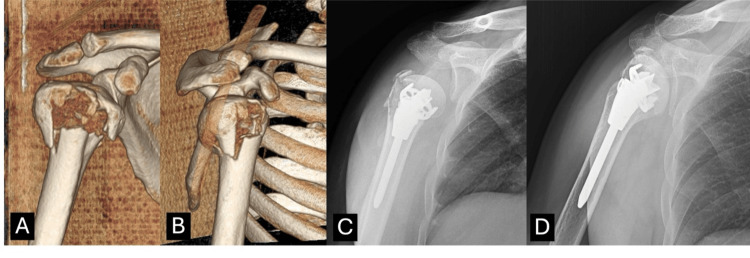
GT resorption after JUST UNIC fixation. (A, B) Preoperative CT images showing the fracture pattern. (C) Postoperative X-ray showing intact GT. (D) Final follow-up X-ray at 12 months showing united fracture with resorbed greater tuberosity. GT - greater tuberosity
